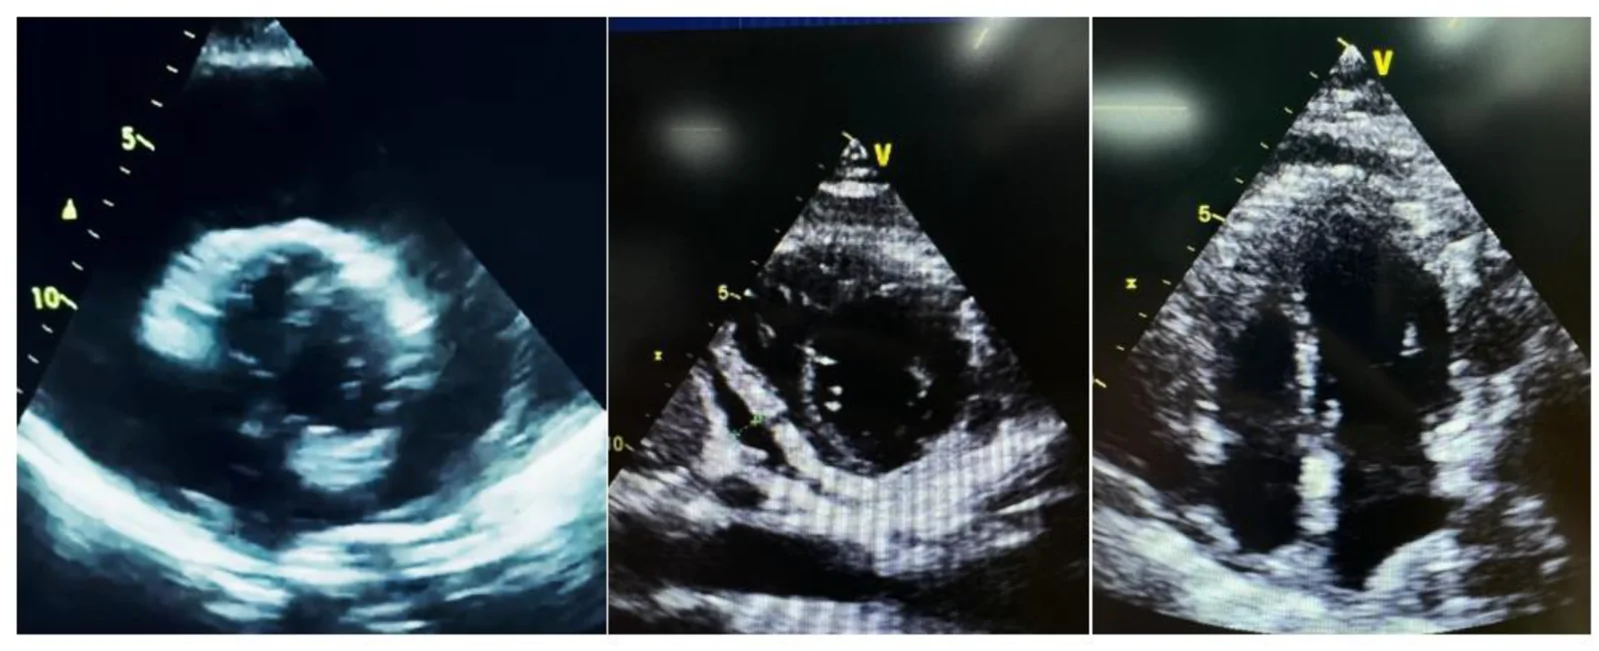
Echocardiography before and after the procedure.
